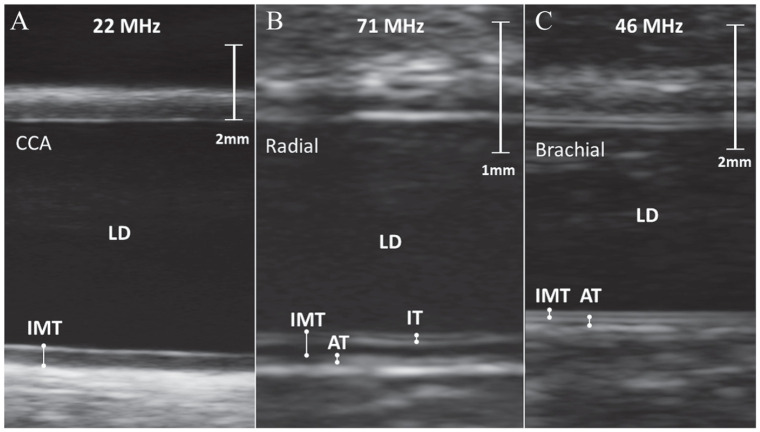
Sample images obtained with ultra-high frequency ultrasound of A) a common carotid artery, B) a radial artery with 4-line intimal thickening and C) a brachial artery. AT–adventitia thickness; CCA–common carotid artery; IMT–intima-media thickness; IT–intima-thickness; LD–lumen diameter.
